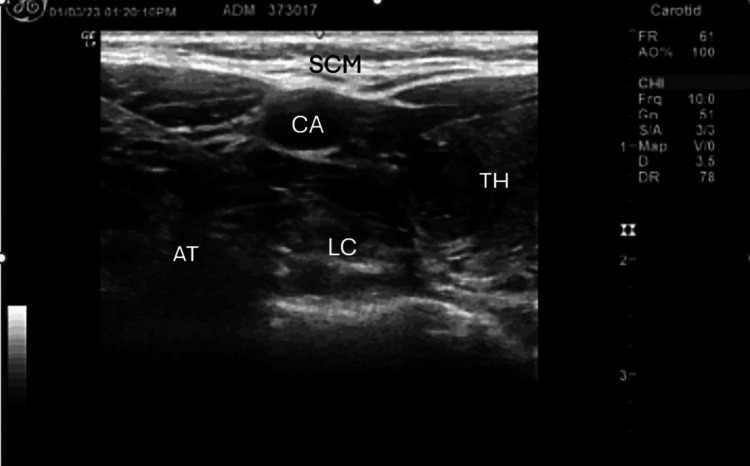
Ultrasound Image of SGB Injection SGB: stellate ganglion block; CA: carotid artery; LC: longus colli muscle; AT: anterior tubercle; TH: thyroid; SCM: sternocleidomastoid muscle
